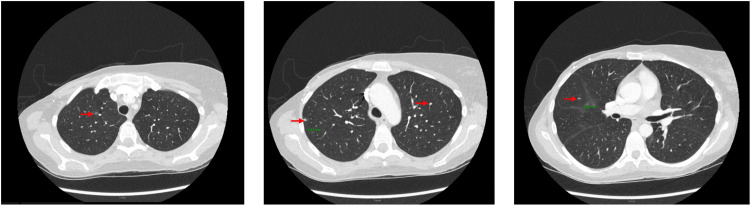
CT chest CT Chest with contrast showing pulmonary nodules throughout the lungs bilaterally, as identified by the red arrows.
